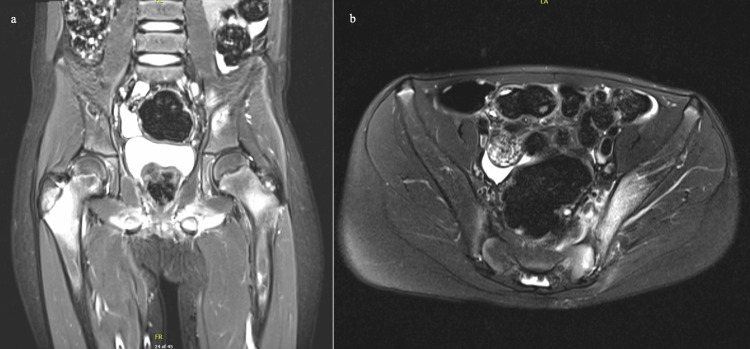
MRI images showing edema-like marrow throughout pelvis and femur
